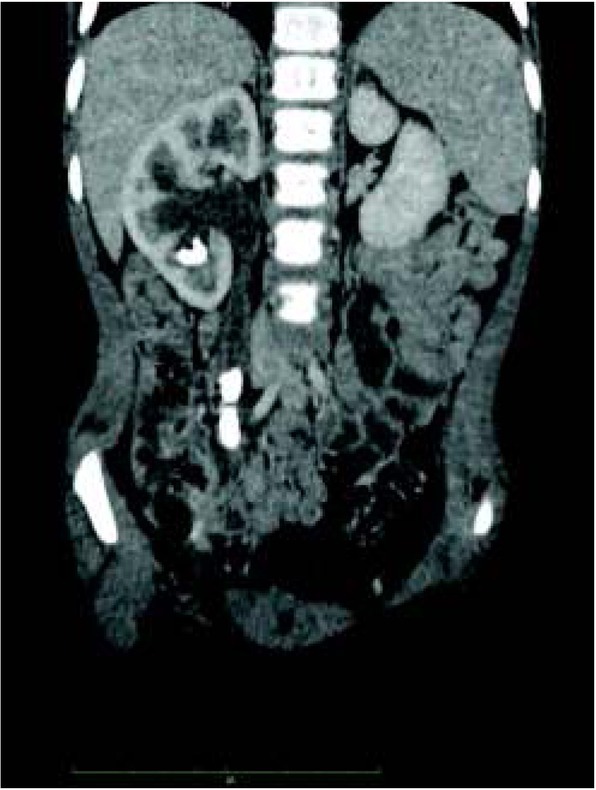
Contrast enhanced CT, corticomedullary phase. Two stones in right ureter and right renal pelvis. Ryc. 3. Tomografia komputerowa ze wzmocnieniem kontrastowym, faza miąższowa. Widoczne złogi w prawym moczowodzie i miedniczce nerki prawej.
